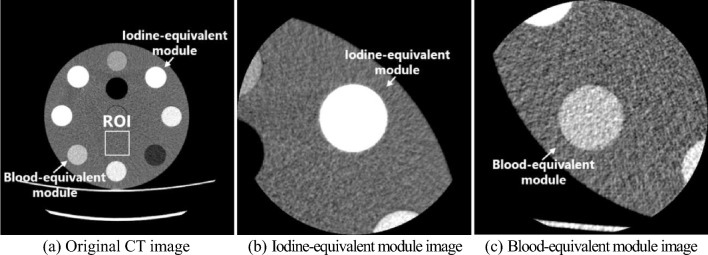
Original CT image and CT images using extended field-of-view reconstruction
